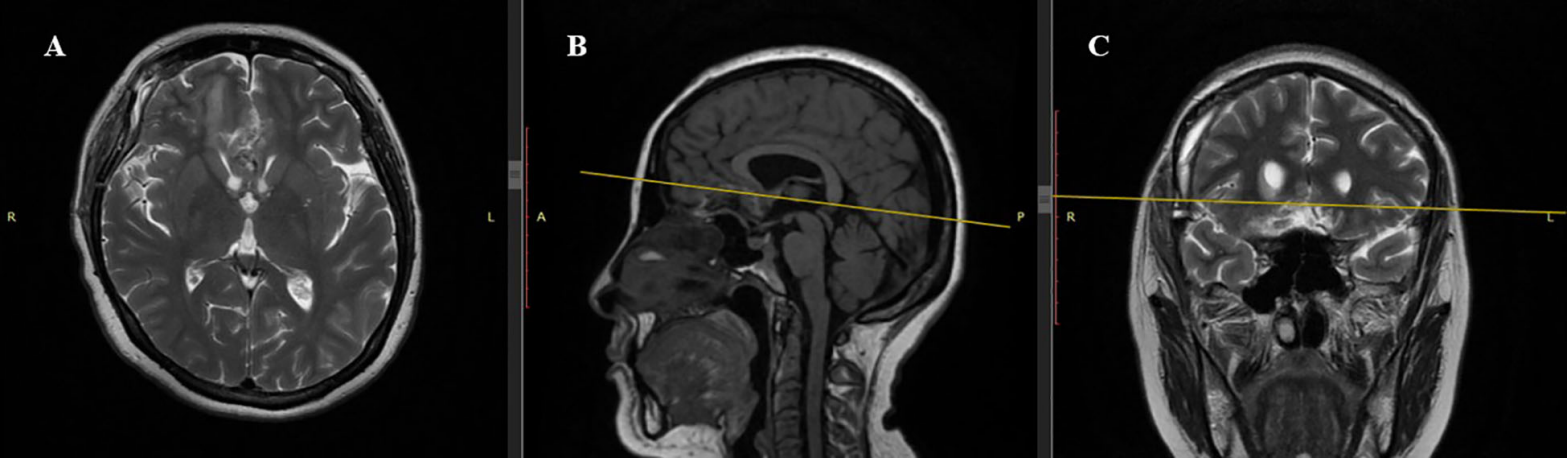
Postoperative MRI Scan (1 month after surgery). (A) Axial T2-weighted sequence: Complete resection of the tuberculum sellae meningioma. Frontal lobes show no significant mass effect. (B) Sagittal T1-weighted sequence: Hypointense resection cavity with no residual tumor. Midline structures, including the corpus callosum, are realigned without compression of basal cerebral structures. (C) Coronal T2-weighted sequence: Hyperintense resection cavity with restored symmetry of frontal lobes and optic chiasm free of compression. No postoperative complications such as hematoma or cerebrospinal fluid accumulation.
